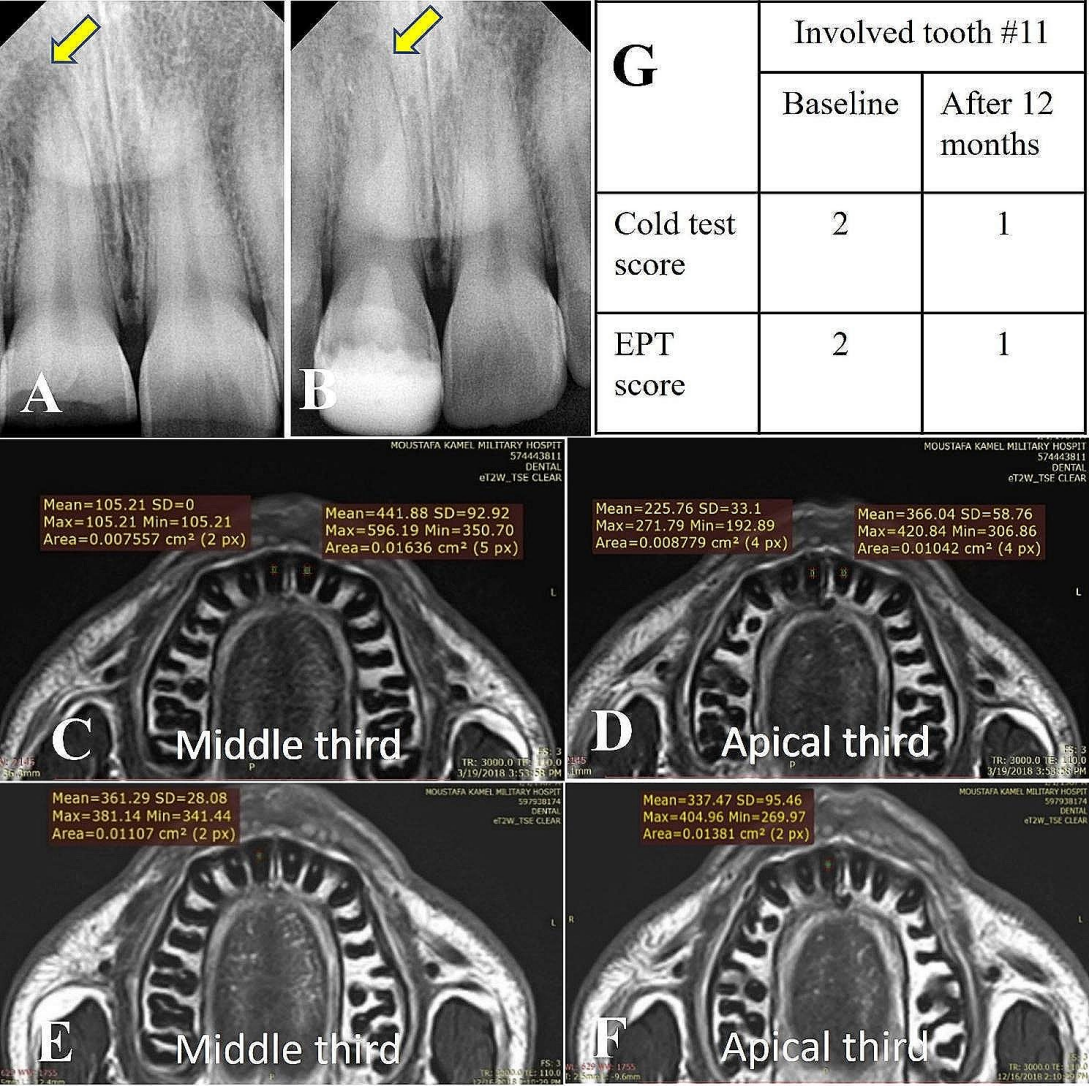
Radiographic images of another representative case: (A) Digital preoperative radiographs with periapical lesions related to tooth #11 (yellow arrow). (B) After 12 months follow-up showing periapical healing (yellow arrow). (C)(D)(E)(F) MRI signal intensity measurements (C) and (D) Middle and apical thirds baseline for both involved tooth (#11) and normal contralateral (#21) (E) (F) Middle and apical thirds after 12 months follow-up period. (G) table representing the cold test and EPT scores for the involved tooth #11
